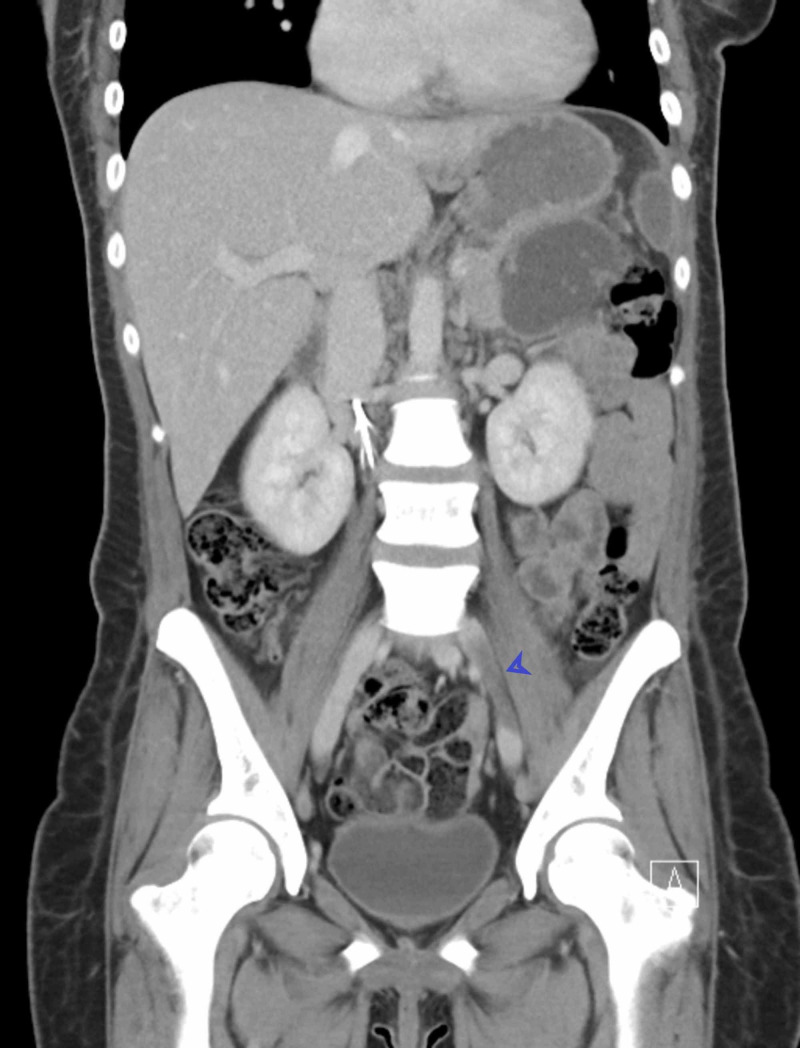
Extension of thrombosis can be seen in CT scan
